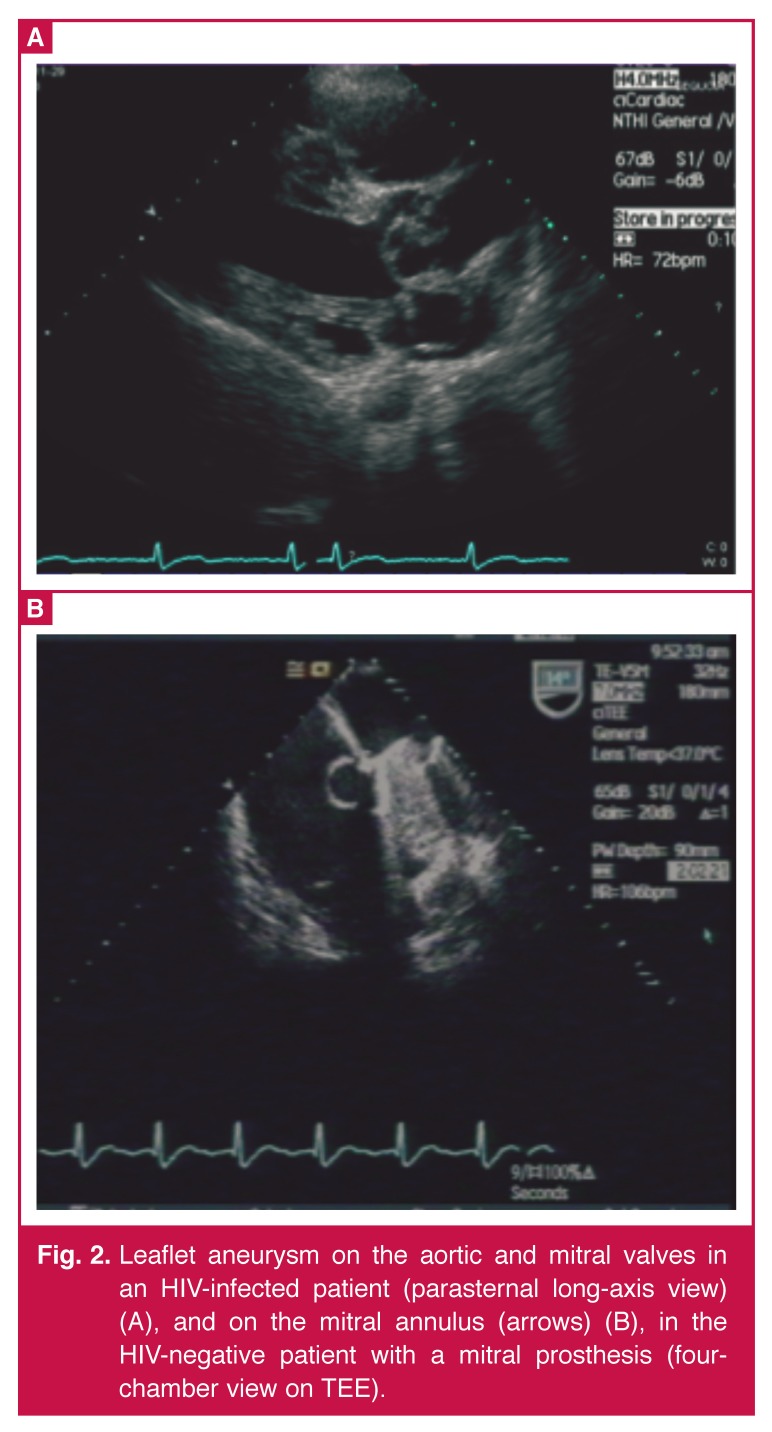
Leaflet aneurysm on the aortic and mitral valves in an HIV-infected patient (parasternal long-axis view) (A), and on the mitral annulus (arrows) (B), in the HIV-negative patient with a mitral prosthesis (four-chamber view on TEE).
